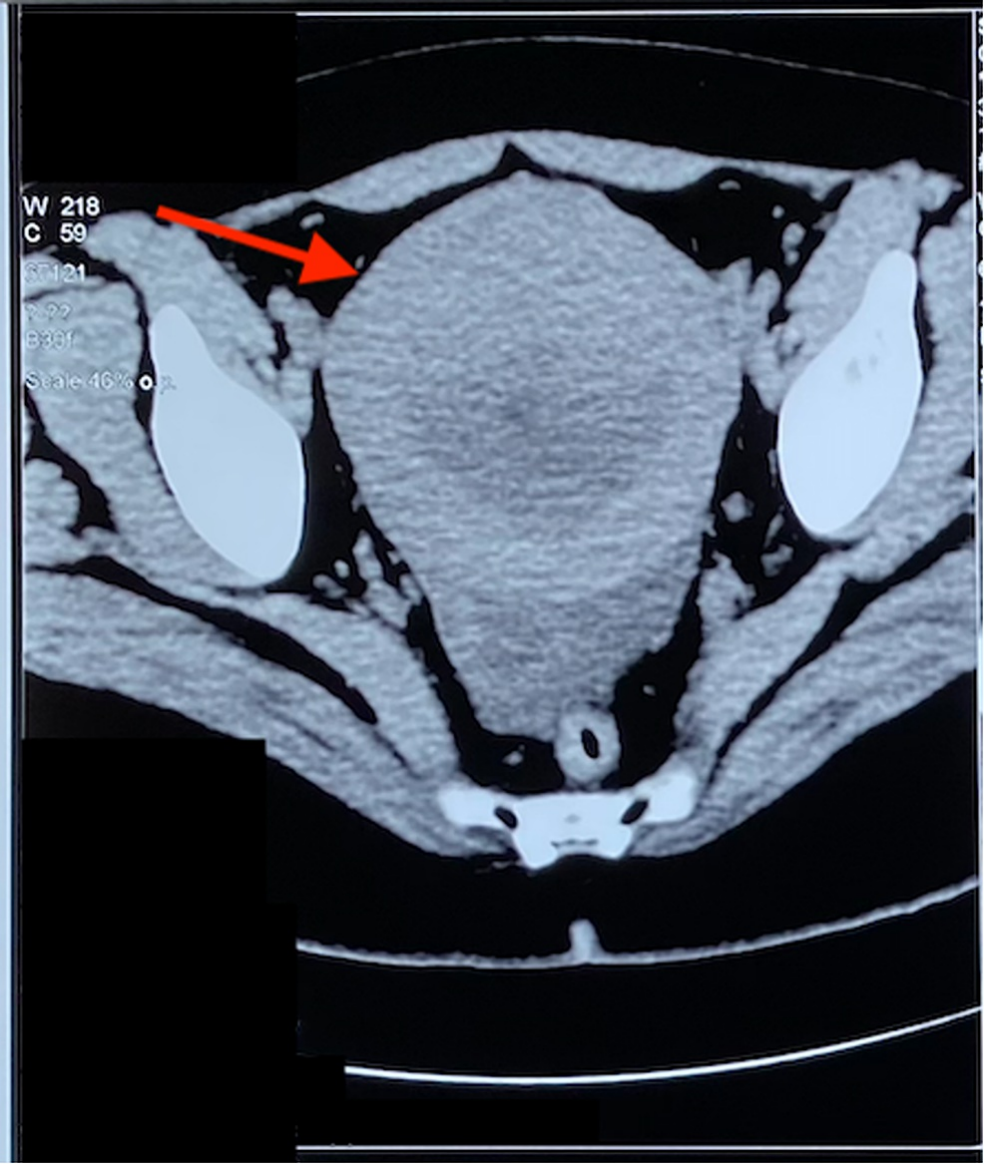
Axial CT scan of pelvis demonstrating an enlarged uterus of 113 mm/ 105 mm/ 135 mm. CT: computed tomography
